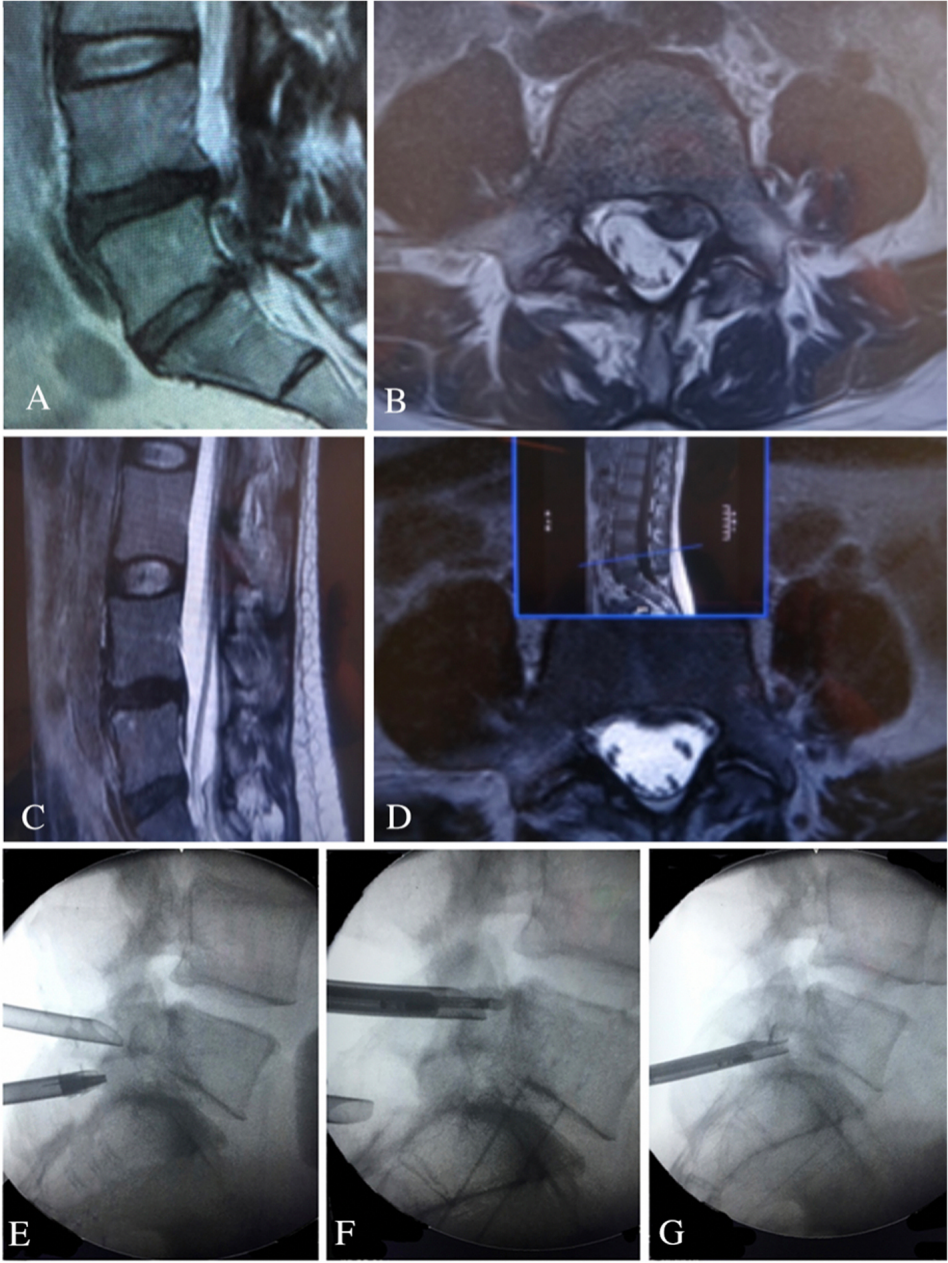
L4–L5 and L5–S1 interlaminar full-endoscopic lumbar discectomy (IELD) for L4–L5 high-grade downward migrated disc at zone 4 due to difficulty reaching the migrated fragment from L4–L5 IELD. a Preoperative MRI lateral view showing L4–L5 high-grade downward migration. b Preoperative MRI axial view showing L4–L5 herniated disc. c Postoperative MRI lateral view showing removal of the migrated disc. d Postoperative MRI axial view showing the removal of the migrated disc. e Intraoperative fluoroscopic view of the two working channels of the double IELD approach. f Intraoperative fluoroscopic view showing IELD from the L4–L5 interlaminar window for L4–S1 zone 4. g Intraoperative fluoroscopic view showing IELD for L5–S1 zone 1
